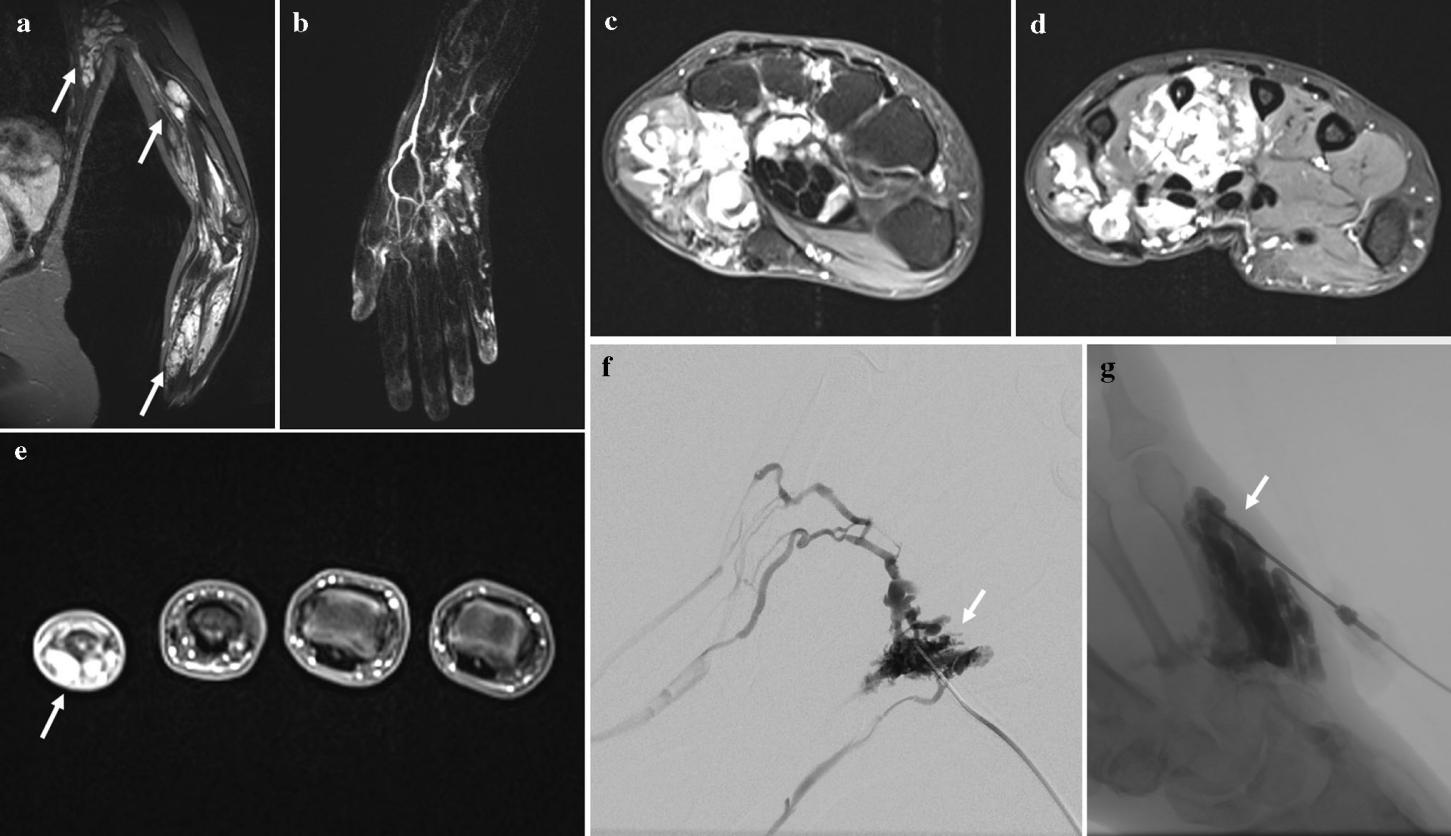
29-year-old female patient with extensive findings of VMs in form of multifocal lesions affecting the left upper extremity. a Coronar T1-weighted fat-saturated fast spin-echo MR image demonstrates three separated hyperintense masses of tubular structures (arrows) extending into the flexor muscles of forearm and humerus and the latissimus dorsi muscle. b-d Time-resolved 3D MR angiography and T1-weighted fat-saturated contrast-enhanced MR images show the inferior venous malformation with extension from the middle of the forearm up to the metacarpalia. e T1-weighted fat-saturated contrast-enhanced MR image reveals involvement of the fifth finger (arrow). f + g Negative roadmap images demonstrate drainage of a lesion (arrow) in normal veins corresponding to type II (Puig’s classification) and filling of another lesion within the wrist and the hypothenar region of the right hand (arrow) without venous drainage
